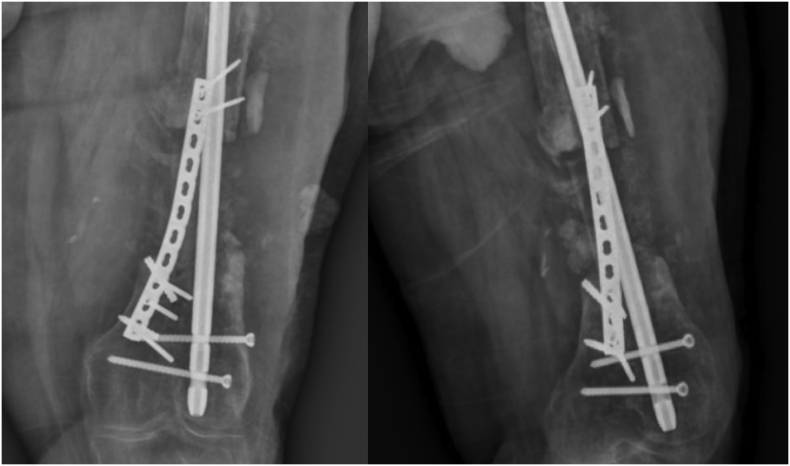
Postoperative radiograph following free fibular graft implantation.
